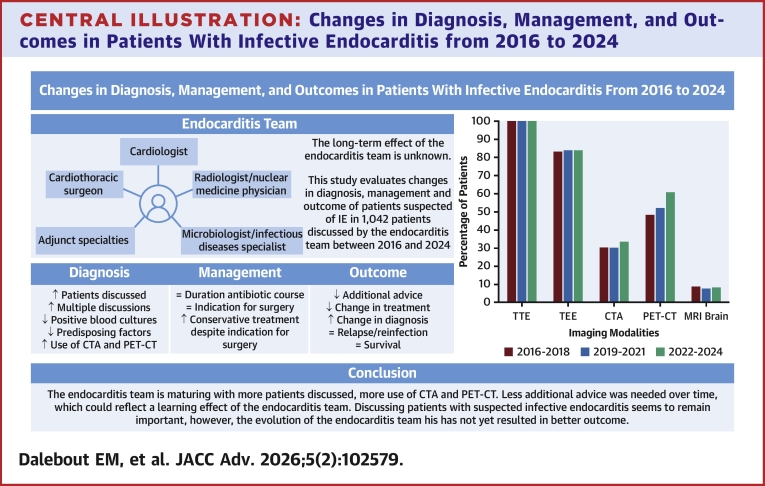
Changes in Diagnosis, Management, and Outcomes in Patients With Infective Endocarditis from 2016 to 2024 Evolution of diagnosis, management, and outcomes of patients with (suspected) infective endocarditis in the 9 years after introducing the endocarditis team. CTA = computed tomography angiography; IE = infective endocarditis; MRI = magnetic resonance imaging; PET-CT = positron emission tomography and computed tomography; TEE = transesophageal echocardiography; TTE = transthoracic echocardiography.
